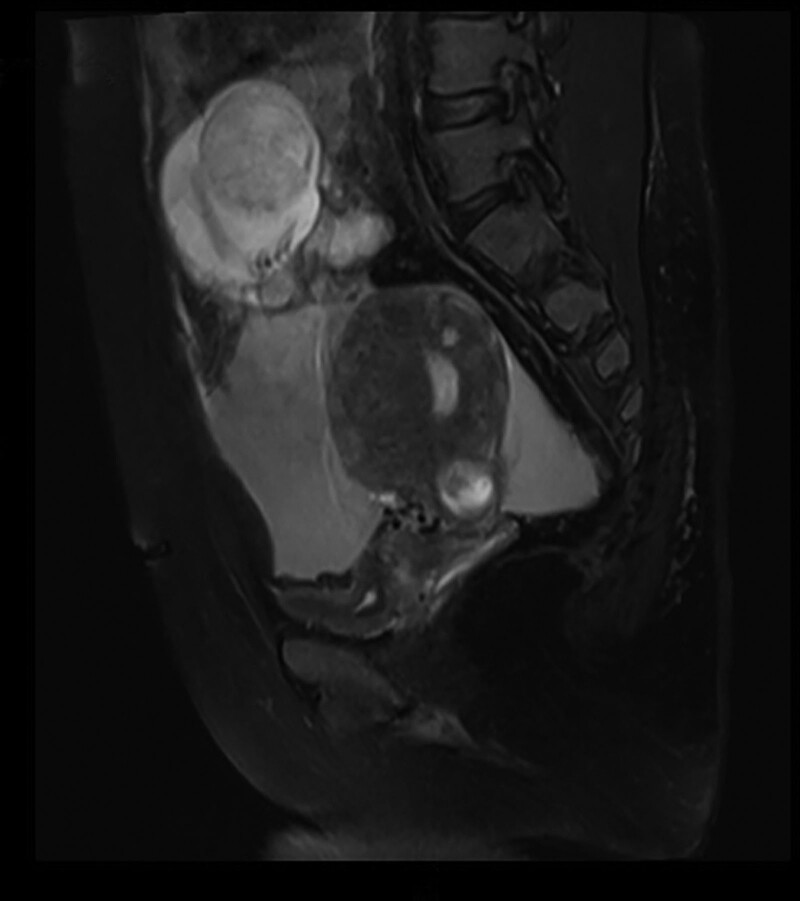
MRI shows an abdominal pregnancy with the fetus above the uterus. MRI = magnetic resonance imaging.
